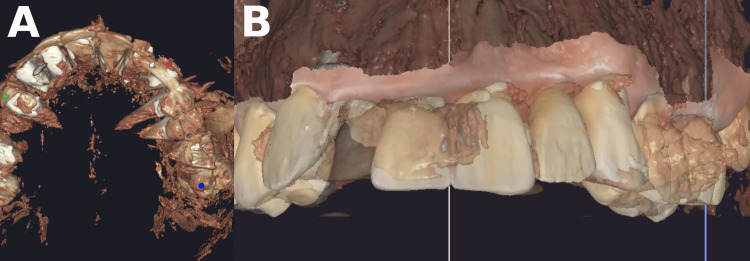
A. CBCT integration into the software program and selection of anatomical anterior and posterior reference markers; B. Superimposition of CBCT-IOS files (frontal view). Proper alignment of incisal edges enhances the registration accuracy CBCT: Cone Beam Computed Tomography, IOS: Intraoral Scanning
